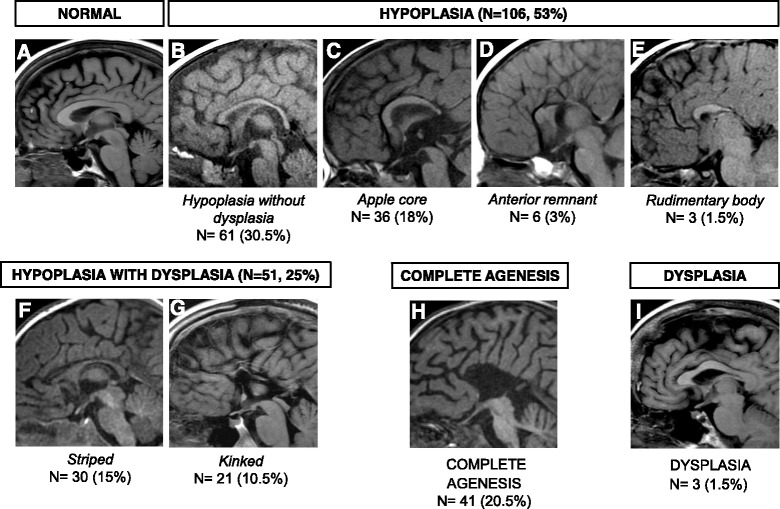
Sagittal MRI images displaying classes and subclasses of corpus callosum abnormalities. Classes of corpus callosum abnormalities denoted in capital letters, subclasses in italics. Number and percentage of patients are displayed for each subclass. a Normal brain (b) hypoplasia without dysplasia (c) apple core (d) anterior remnant (e) rudimentary body (f) striped (g) kinked (h) complete agenesis (i) dysplasia
